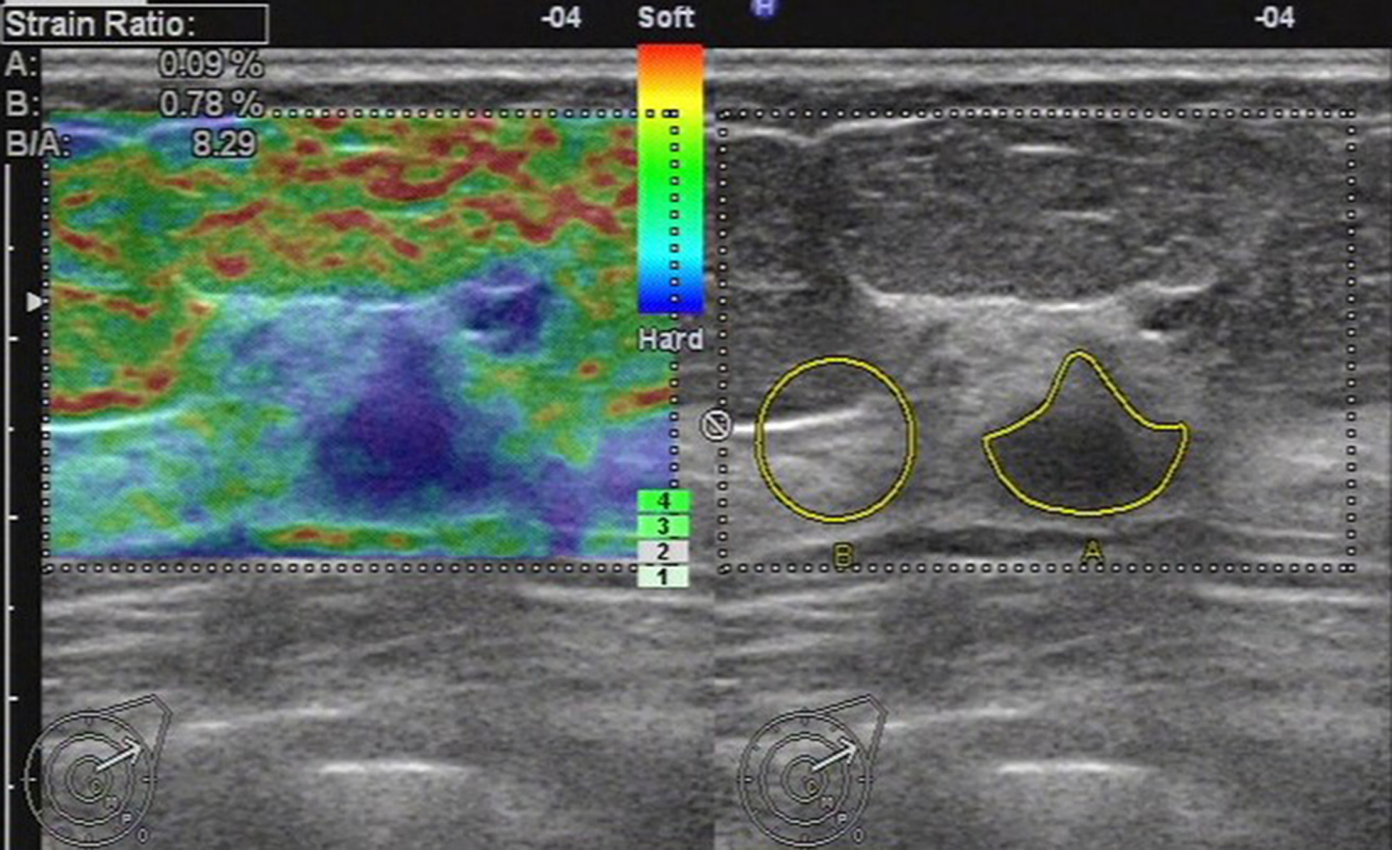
Ultrasonic elastographic image of an invasive lobular carcinoma in a 77-year-old woman. The lesion was scored 4 with the 5-point scoring method. The strain ratio was 8.29 with the strain ratio method at the same breast tissue depth as the reference.
